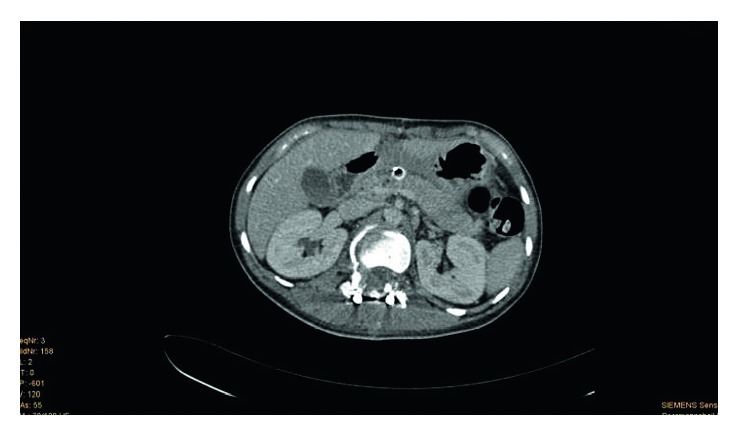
(Patient 3, scan 1) CT scan 19 days after trauma and 17 days after laparotomy due to pancreatitis. Drainage at the level of the upper abdomen placed along the left hepatic lobe of the pancreas head and body. There are discrete air pockets between the left hepatic lobe and the pancreatic head. The pancreas appears to be vigorous and slightly blurred. In the peripancreatic tissue, low-density increases are observed. No new liquid formations are observed.
